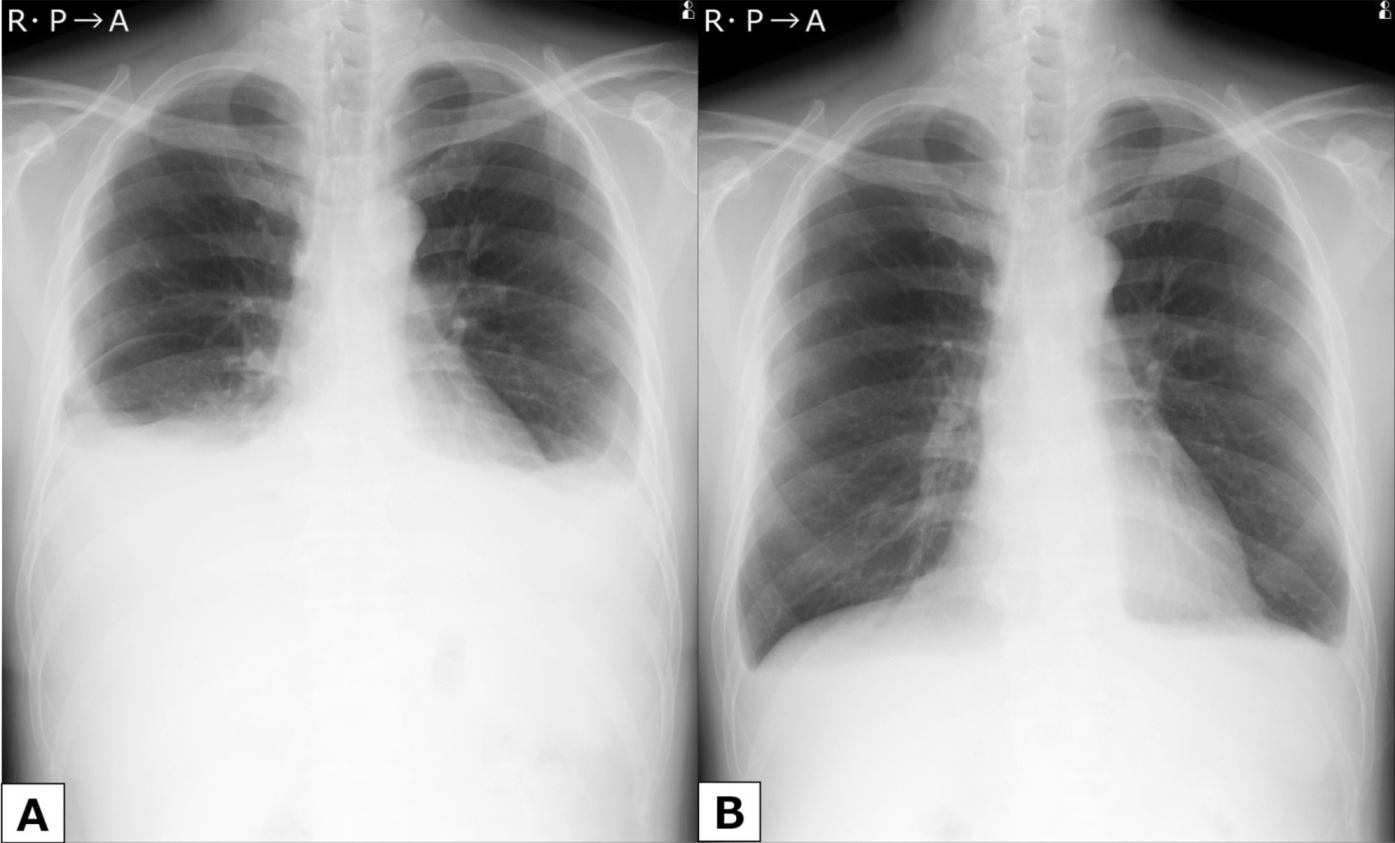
Clinical course of pleural effusion. (A) Chest radiograph at admission, 22 days after the first discontinuation of dasatinib. (B) Final follow‐up chest radiograph obtained 239 days after the initial discontinuation of dasatinib showed marked improvement of bilateral pleural effusions.
